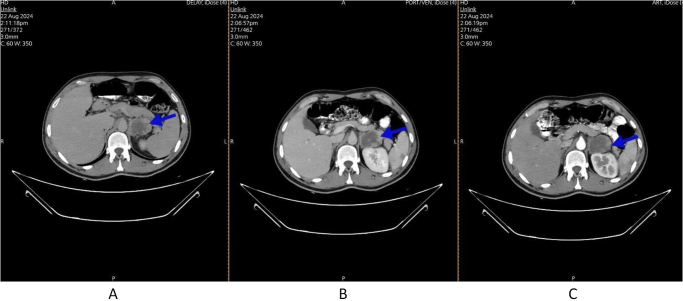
Axial view of CT scan with IV contrast was done and showed the following: a Left adrenal mass measuring about (4.8 × 4 cm), with heterogeneous progressive enhancement post contrast administration (blue arrows): (A) delayed phase, (B) venous phase, and (C) arterial phase.
